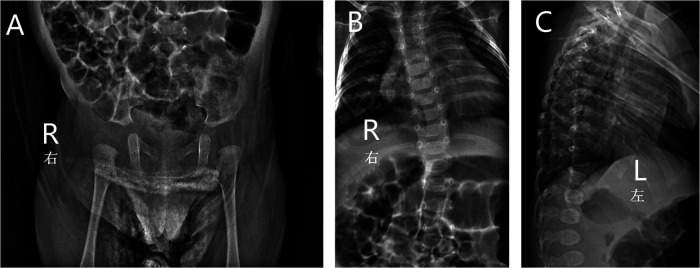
X-ray of the patient showed widened right hip joint space (A), spinal deformity, dislocation (B) and slight kyphosis (C).
